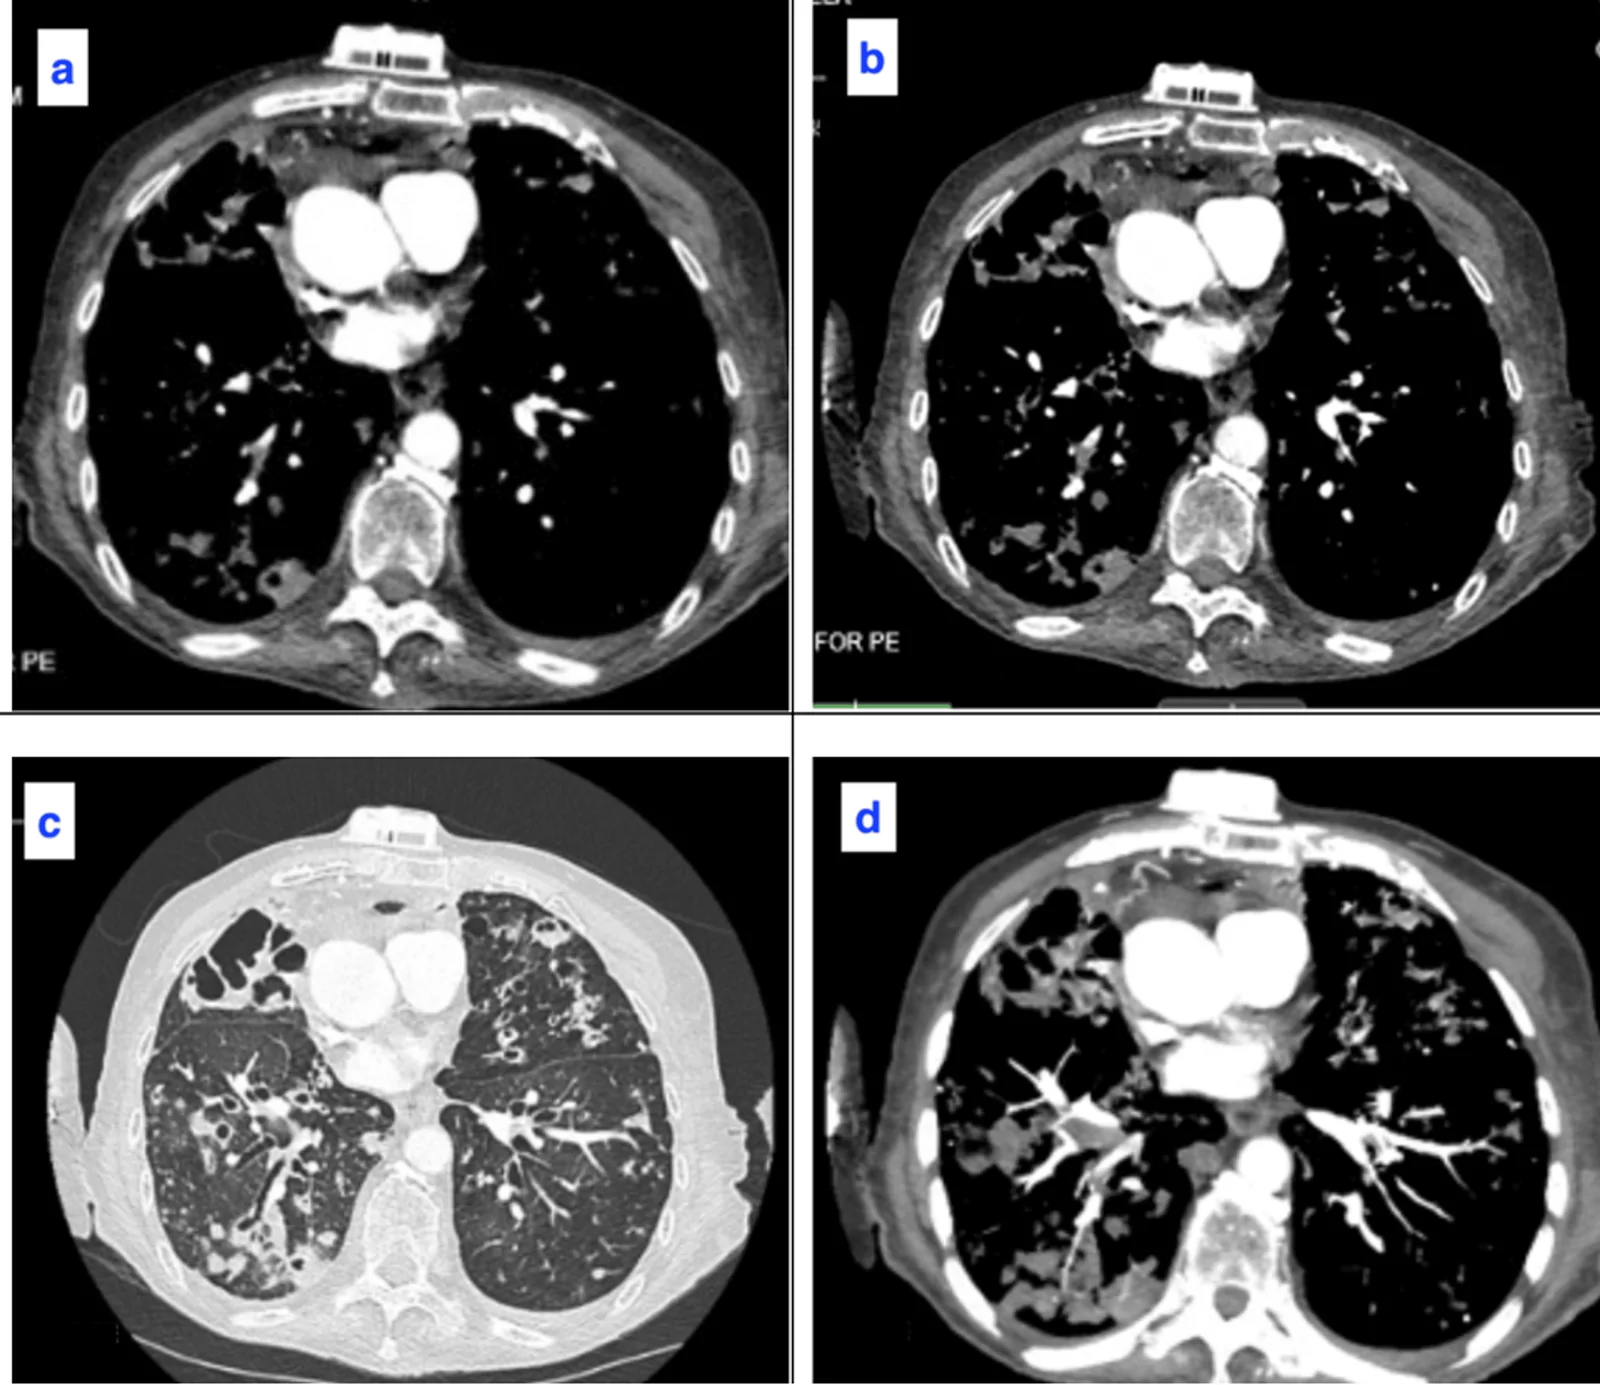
Contrast-enhanced computed tomography (CT) of the chest obtained at admission. (a–d) Axial CT images demonstrating extensive bilateral cavitary pulmonary lesions, most pronounced in the right lung, with surrounding ground-glass opacities. Numerous bilateral pulmonary nodules, areas of consolidation, and bilateral bronchiectasis are also present. No pleural effusion or pneumothorax is identified.
